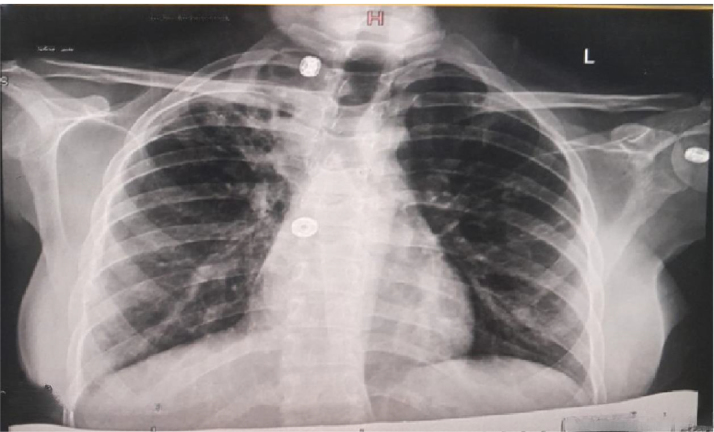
Chest radiograph demonstrating inhomogeneous opacification with cystic lucencies in the right upper lung zone, consistent with bronchiectatic changes.
